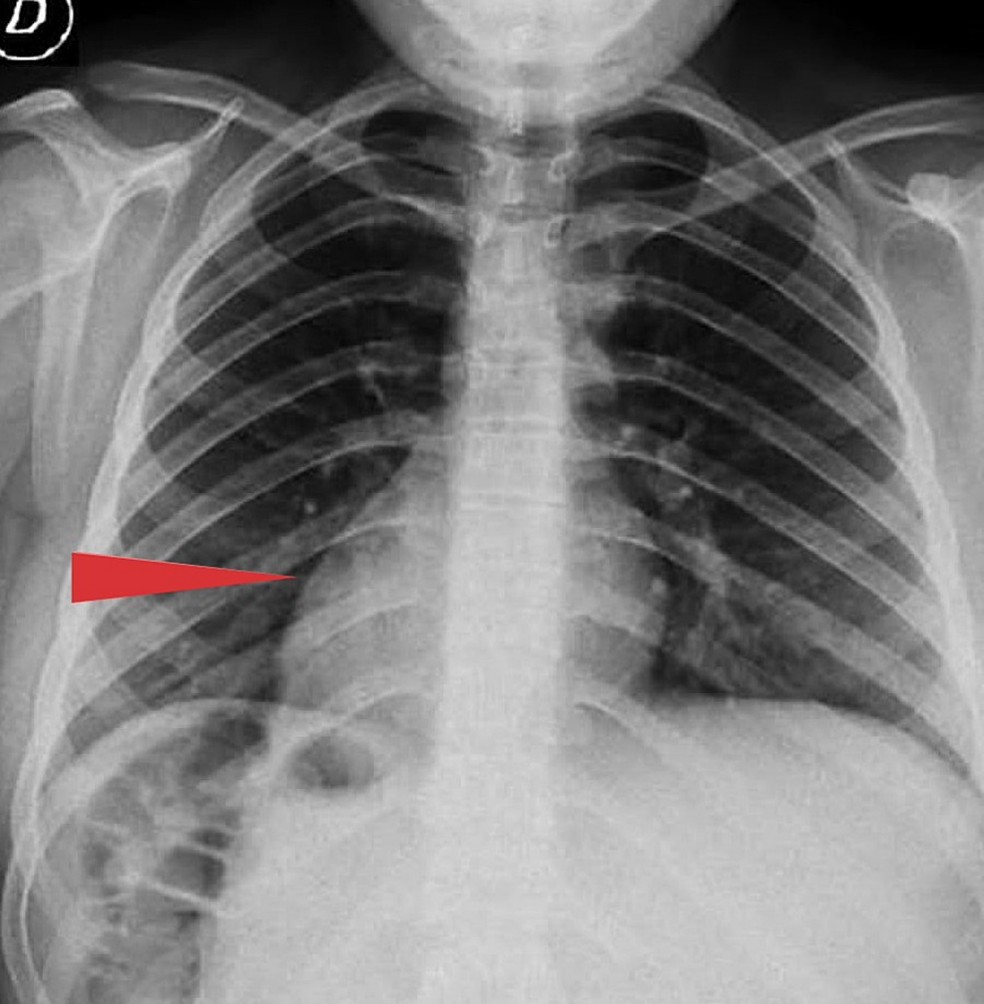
Chest radiograph showing dextrocardia
